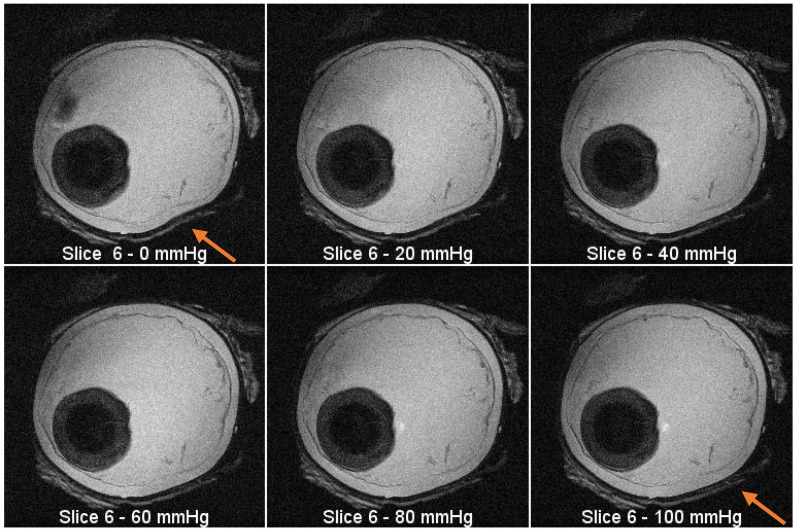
MRI images from the same slice (plane) at increasing pressure intervals. There is visible expansion in the outline of the eyeball as the pressure increases (see arrow location).
